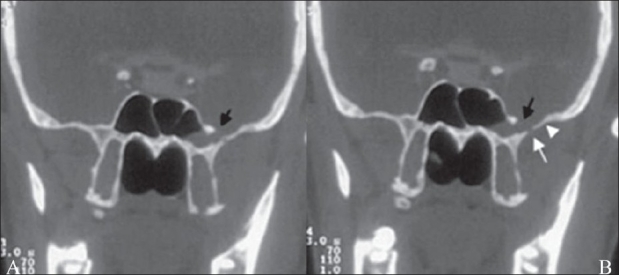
Coronal CT cisternography displays an approximately 3-mm size bony defect in the left lateral wall of the sphenoid sinus near its junction with the greater wing of the sphenoid (black arrow) along with a second, smaller, bony defect located laterally in the greater wing of the sphenoid on the left side (white arrow in B). Remodeling of the greater wing is also shown, which, as compared with the normal right side, is thinned out and bulges toward the infratemporal fossa (arrowhead in B)
